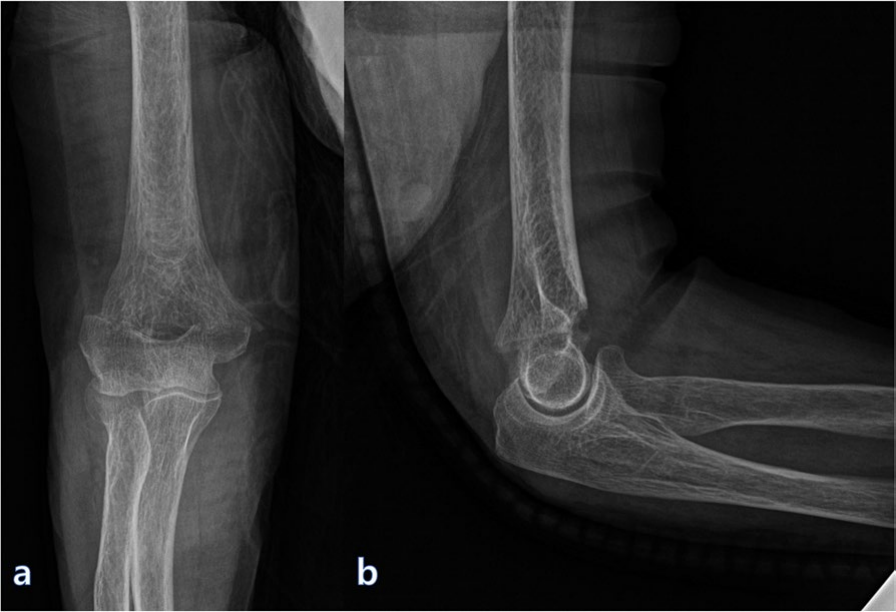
a Anterior–posterior and b lateral radiographs of a 75-year-old female patient taken before surgery. The fracture was defined as an extra-articular fracture with a single transverse fracture line that consistently exited at the level of or distal to the lateral epicondyle laterally and at the level of or just proximal to the medial epicondyle
